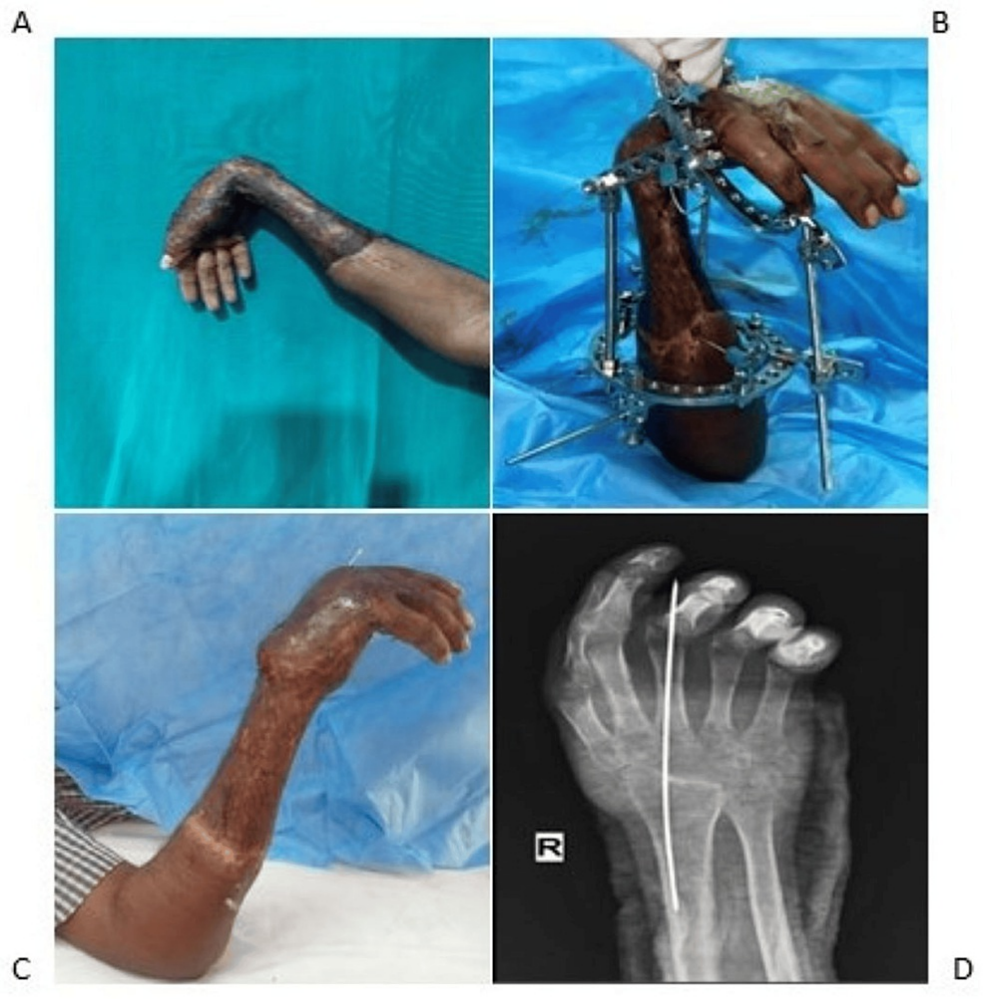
Post-traumatic wrist flexor contracture deformity of patient 3 (A). Patient 3 underwent right wrist Ilizarov ring fixator application (B). Correction of wrist flexion deformity postoperatively (C). Postoperative X-ray of the right wrist after the carpectomy (D).
